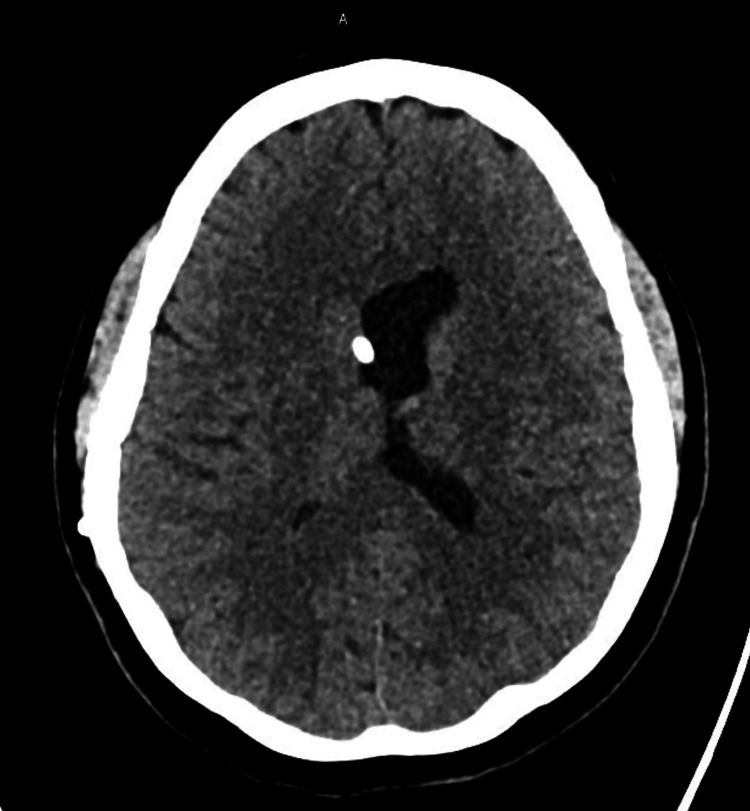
Noncontrast CT brain This figure shows a noncontrast CT of the brain in September 2025, demonstrating the stable position of the patient's VP shunt in the lateral ventricle. CT: computed tomography, VP: ventriculoperitoneal
